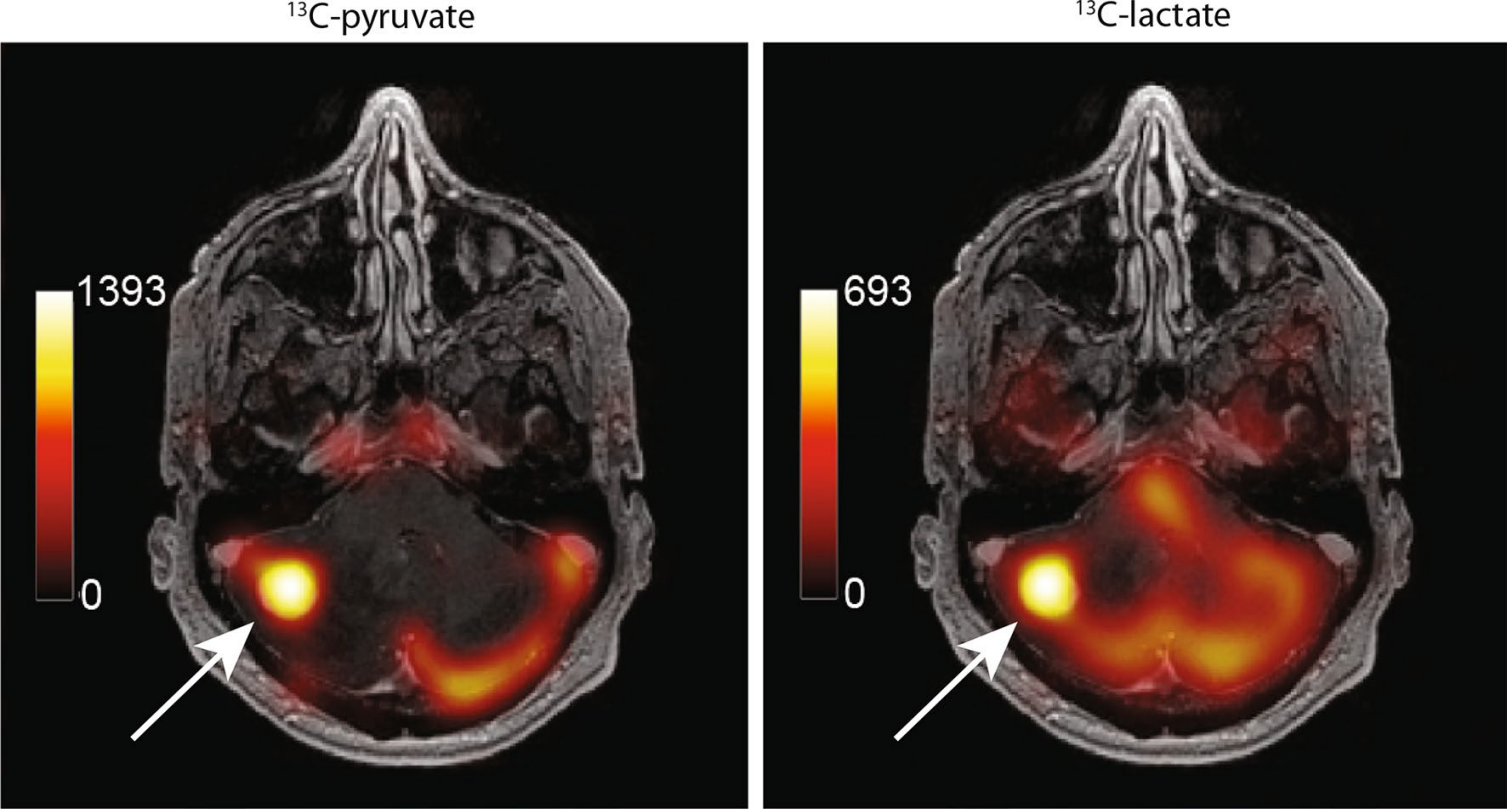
Example $$^{13}$$C-metabolite images of an intracranial metastasis (arrows) in a renal cell carinoma (RCC) patient. The metabolite signals are displayed as colour overlays on the corresponding greyscale T1-weighted anatomical images, and were computed by summing 12 time-points collected over a 60 s acquisition window. The treatment of this high-lactate lesion with SRS failed, with local progression prior to the 6-month followup
